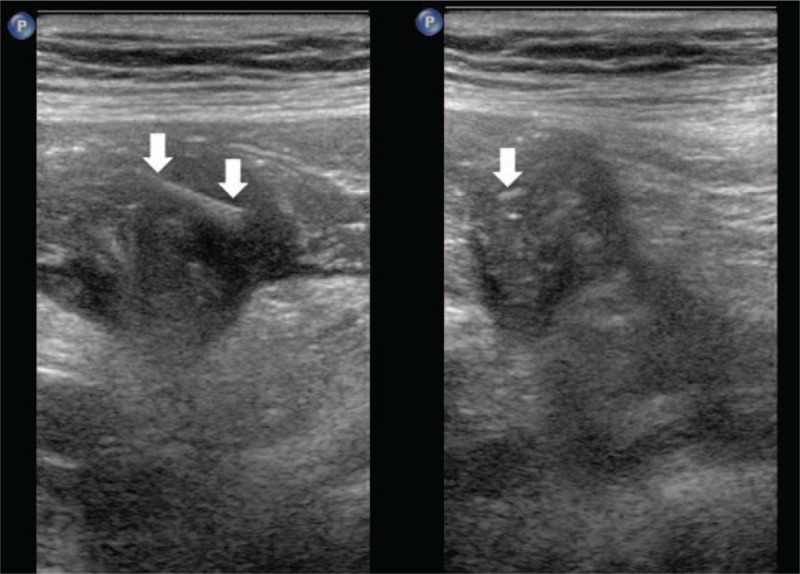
Fish bone perforating small intestinal on ultrasound images. An ingested foreign body (arrow) presumed to be a fish bone is incidentally seen in the small intestinal on these ultrasound images. Ultrasound images demonstrating a linear echogenic (short axis on the right, long axis on the left) foreign body (arrows).
